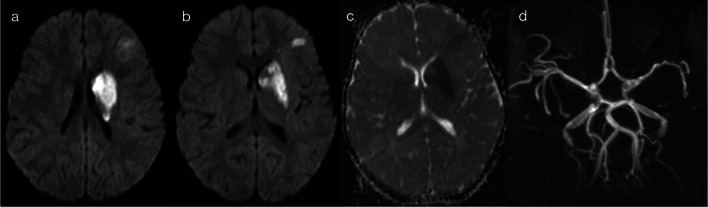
Restricted diffusion in supratentorial grey matter—vascular territory infarct (Pattern 2C). 2-year-old child with right upper and lower limb weakness in the setting of prior varicella infection (IgG positive), in keeping with post-varicella arteriopathy. DWI (a, b) and apparent diffusion coefficient (ADC) map (c) were consistent with an acute left caudato-lenticular infarct and a small infarct within the left frontal lobe. MR angiography (d) demonstrated left middle cerebral arteriopathy
